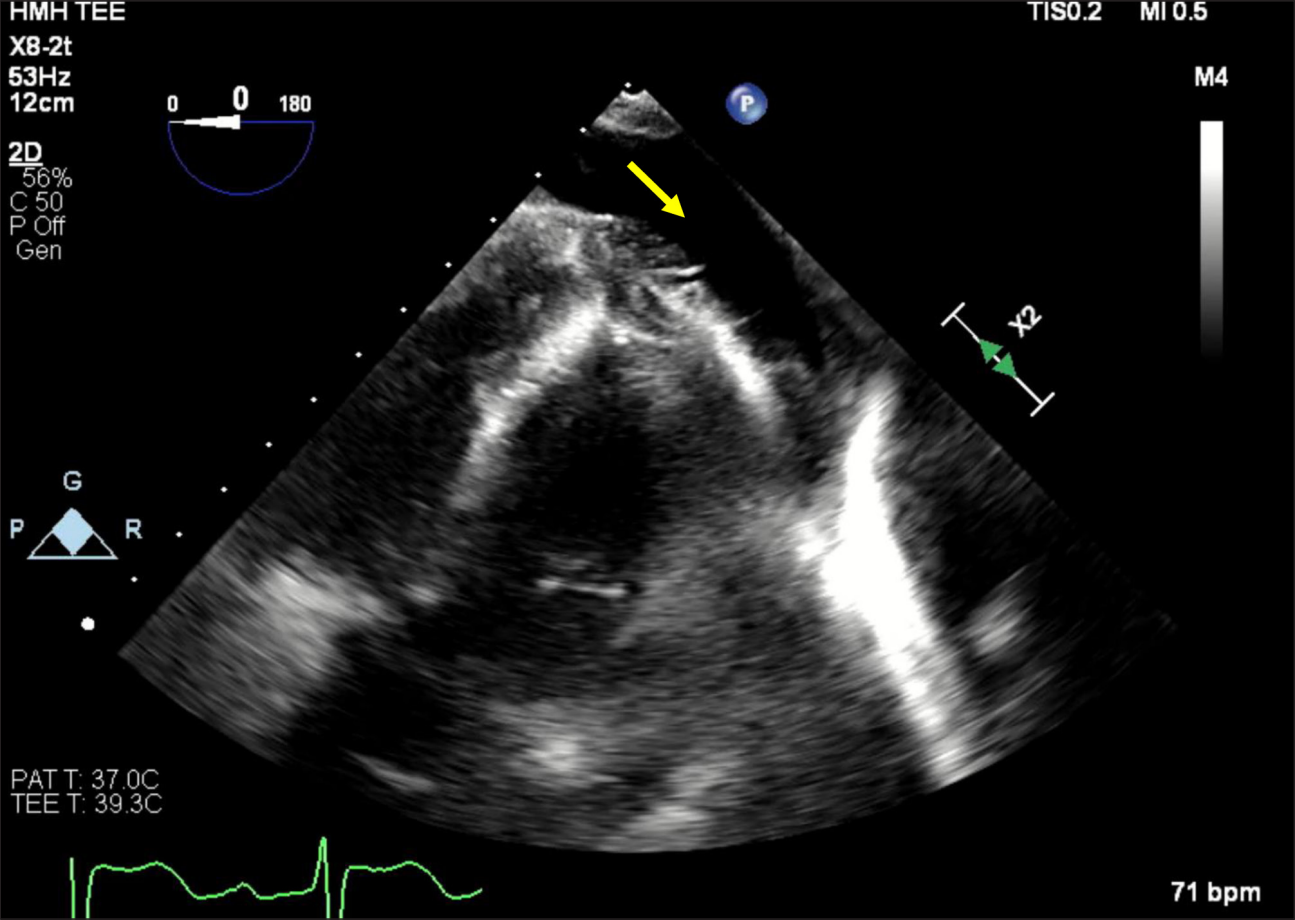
Transesophageal echocardiogram, transgastric view showing moderate pericardial effusion (yellow arrow) during MitraClip procedure that required urgent pericardiocentesis. See Figure 5 videoclip at https://youtu.be/_4LF1h95oR0.
